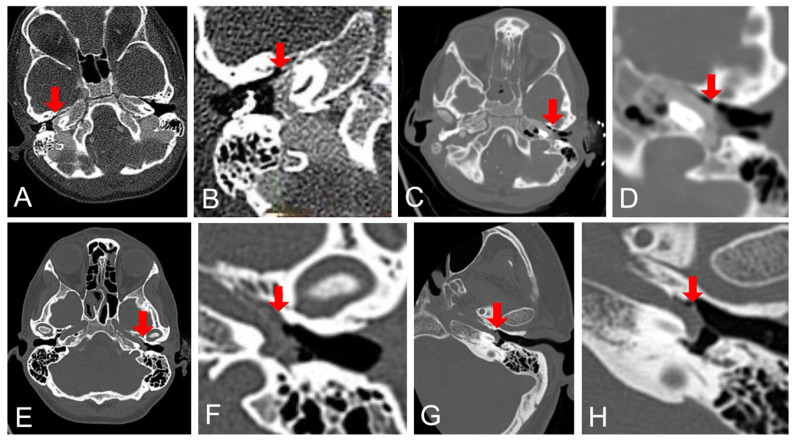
Axial CT temporal bone imaging. Overview and close-up of the tympanic cavity of case 1 (A,B), case 2 (C,D), case 3 (E,F), and case 4 (G,H), respectively. All aICAs, each with a similar course through the tympanic cavity in a dorsal to anteromedial direction, lateral to the cochlea in front of the promontory, are marked with a red arrow.
